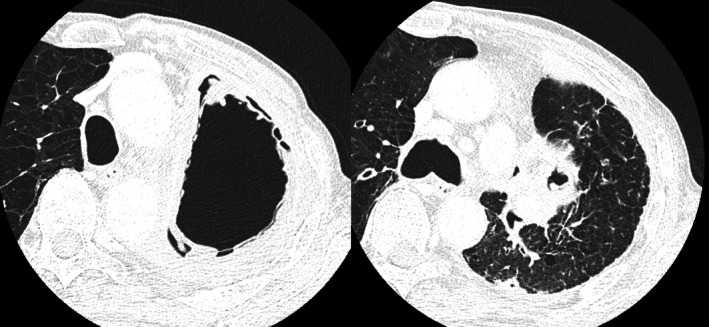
Chest computed tomography demonstrating a postoperative thoracic cavity in the upper left hemithorax with irregular inner wall thickening and communication between the bronchus and the cavity.
